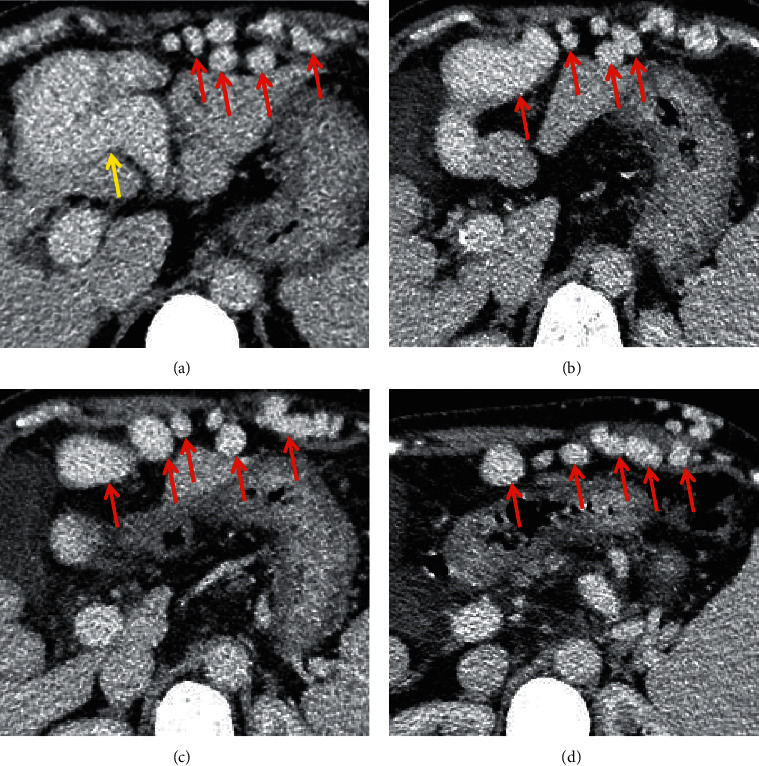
Recanalized paraumbilical vein. In a 65-year-old female with liver cirrhosis, the axial contrast-enhanced CT scan on the portal vein phase demonstrated that the recanalized paraumbilical vein (red arrow) was a round, dilated, or tubular enhanced structure, originating from the left portal vein branch (yellow arrow).
